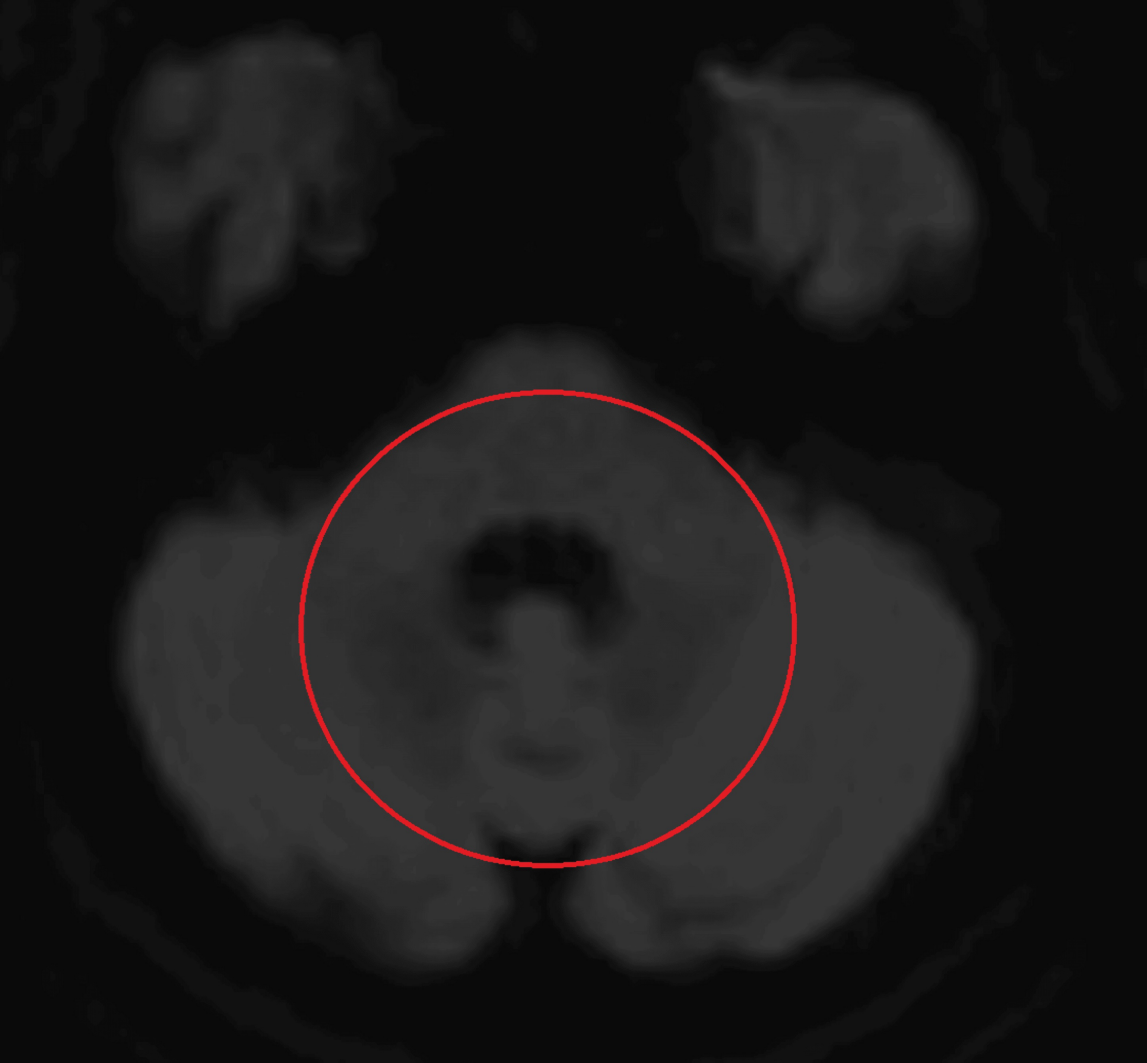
DWI sequence of brain MRI showing no restricted diffusion abnormality in pons or cerebellum. The red circle shows the area of interest. DWI: diffusion-weighted imaging
